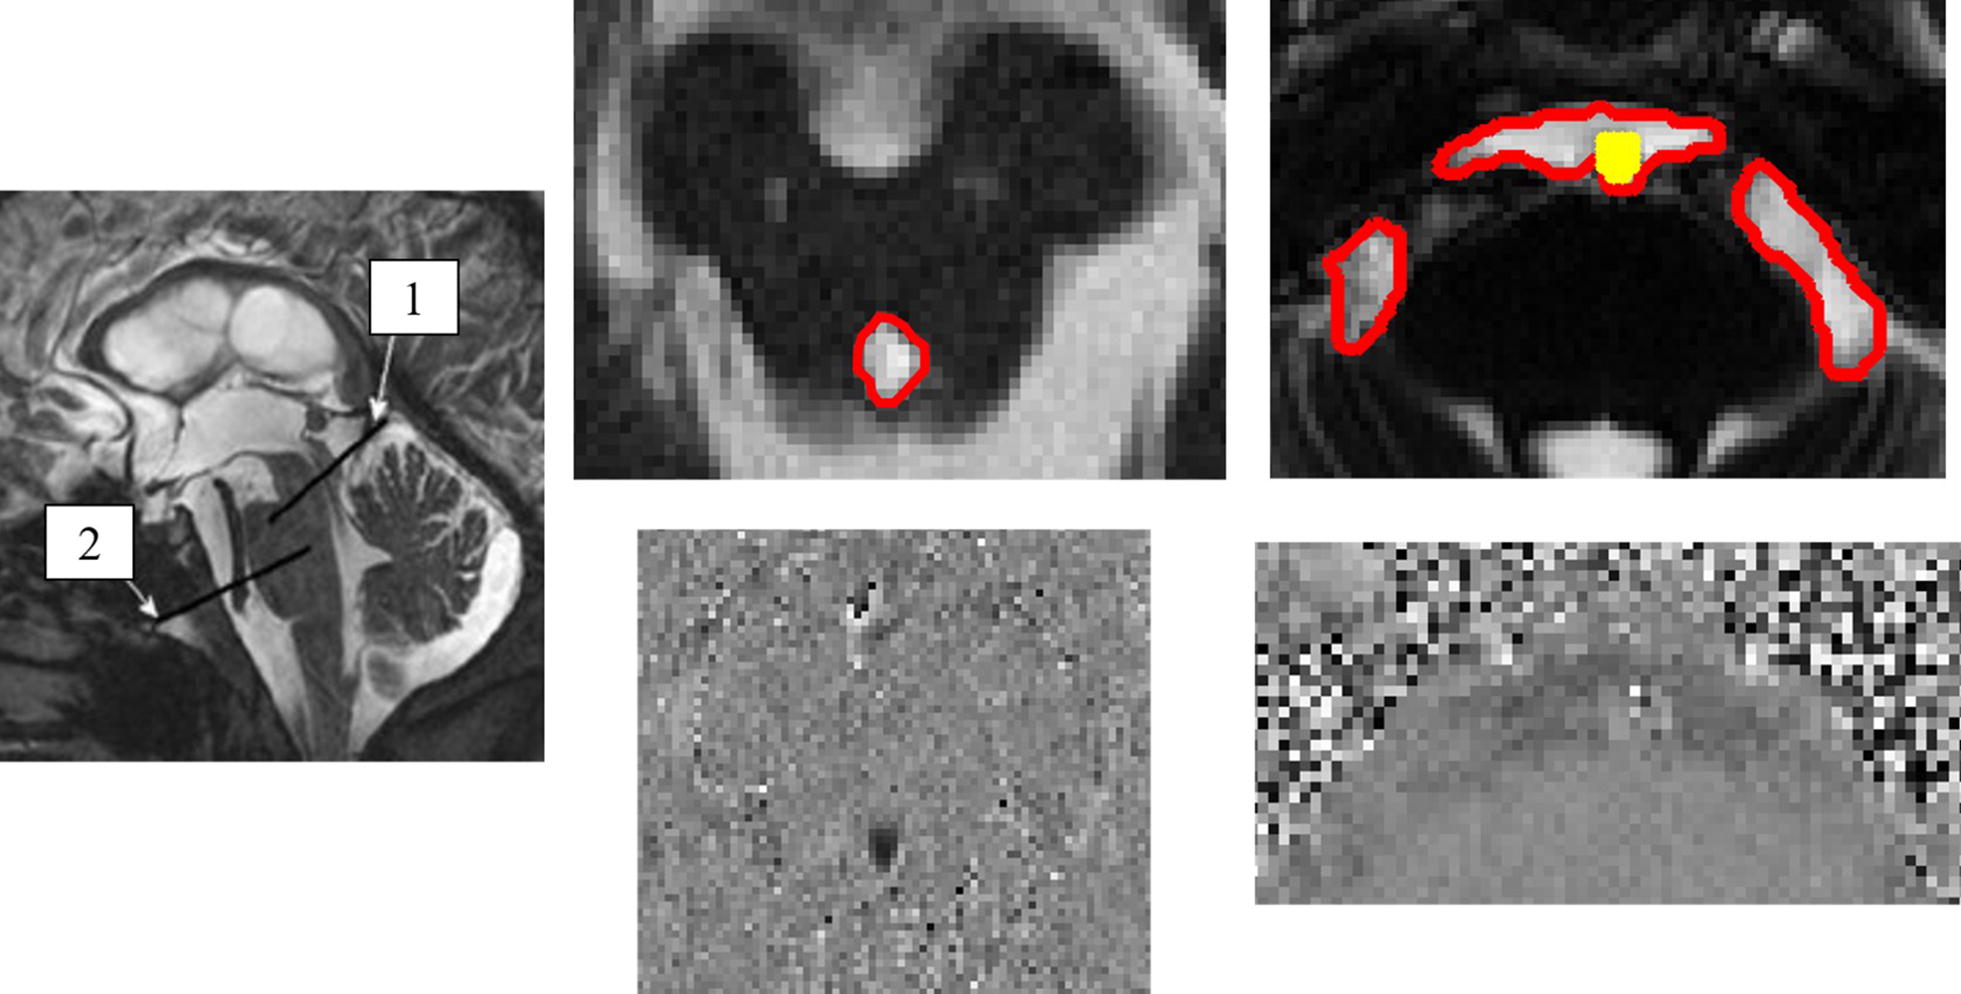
Left) Midsagittal T2-weighted image, flow acquisition planes for (1) cerebral aqueduct and (2) prepontine cistern. Planes were defined perpendicular to CSF flow. Center top) Example of cerebral aqueduct (T2 TruFisp) with the region of interest for the flow quantification outlined in red. Center Bottom) Example of the phase contrast sequence for the cerebral aqueduct during peak caudal CSF flow. Right top) Example of prepontine cistern (T2 TruFisp) with the region of interest for the flow quantification outlined in red and the basilar artery highlighted in yellow. Right bottom) Example of the phase contrast sequence for the prepontine cistern during peak caudal CSF flow
